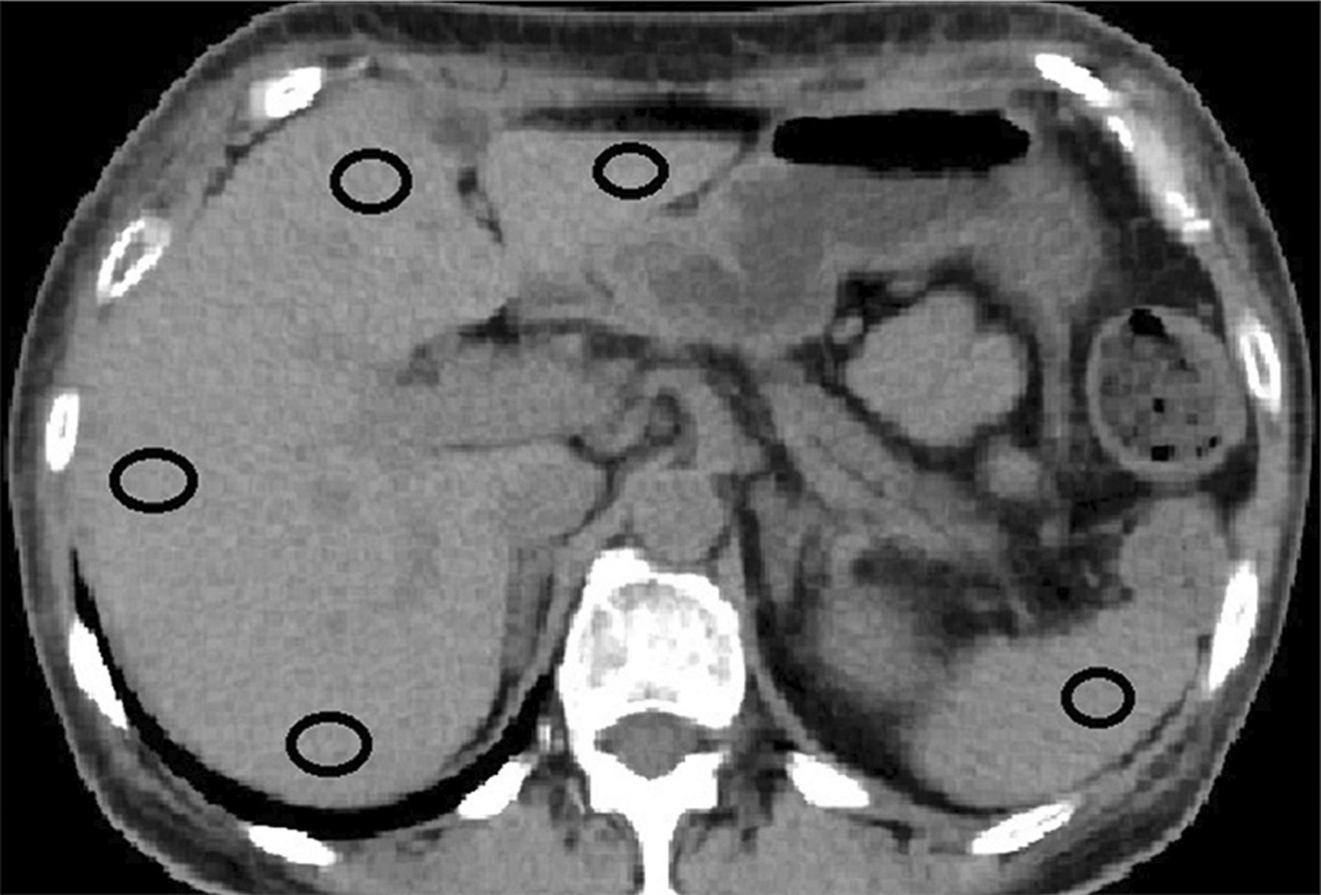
Liver and spleen attenuation values were measured on plain computed tomography images. The degree of liver attenuation was measured in four regions of interest in different sections of the organ. The degree of spleen attenuation was measured in one region of interest in the organ.
